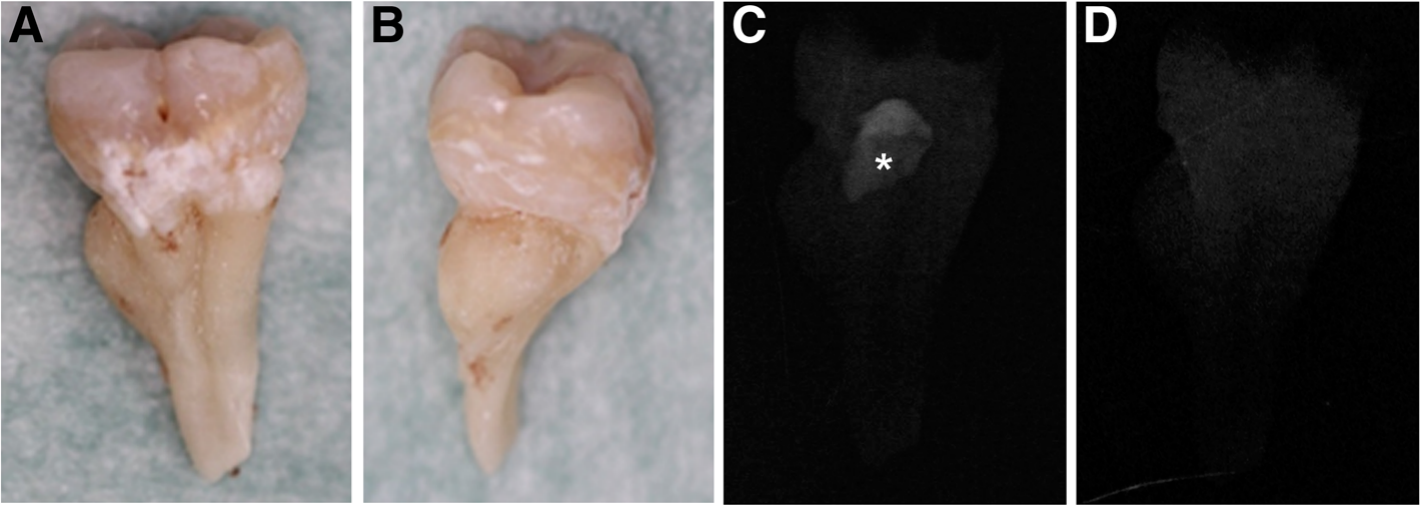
Photographs of the left mandibular first molar (36) taken before the demineralization procedure and progression of the demineralization process as monitored by dental radiographs. Tooth 36 from the (a) buccal and (b) mesial surface. Note the aberrant root morphology and a ribbon of hypomineralized enamel extending circularly around the tooth crown and toward the cervical area. (c) Radiograph of tooth 36 taken 14 weeks after demineralization with the mild bone-decalcification solution Osteosoft®. Note incomplete decalcification with unusual mineralized tissue in the area of the pulp chamber (asterisk). (d) The process was successfully completed after additional overnight decalcification with a 1:1 mixture of Osteosoft® and Osteomoll® solution
